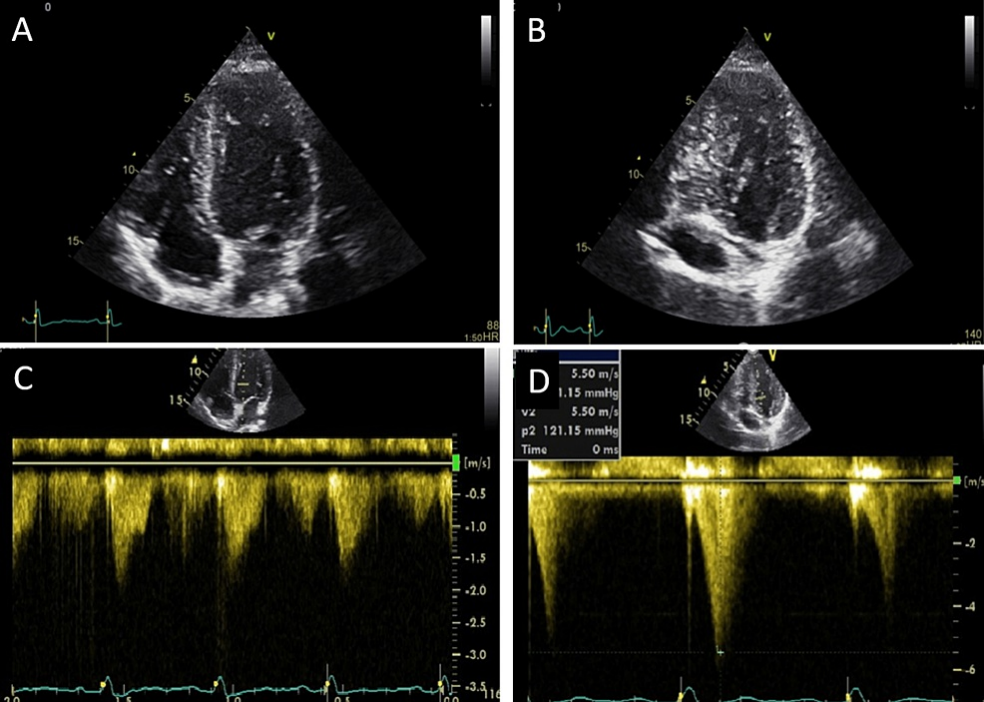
(A) Apical four-chamber before exercise; (B) Apical four-chamber at peak exercise; (C) CW Doppler at rest without significant intraventricular gradient D and at peak exercise with significant intraventricular gradient CW: Continuous Wave
